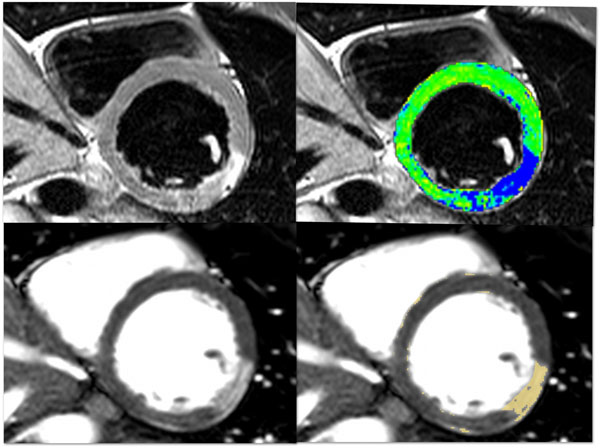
Top left: Short axis T2-weighted dark blood TSE image showed oedema in mid lateral wall of left ventricle. Top right: Short axis T2-weighted dark blood TSE image. Oedema ratio showed oedema in the mid lateral wall (blue) compared to the relative normal myocardium (green). Bottom left: Short axis post-gadolinium SSFP image showed corresponding oedema in mid lateral wall. Bottom right: Short axis post-gadolinium SSFP image highlighted the oedema area by semi-automated software using 2 standard deviation of mean normal myocardium.
